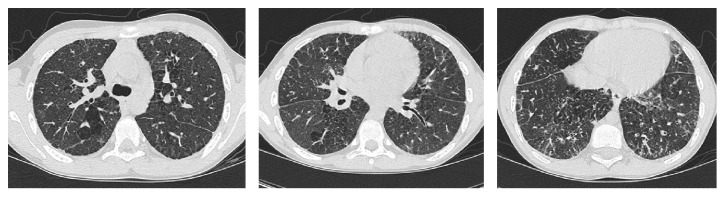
CT of chest showed area of emphysematous changes, several small lung cysts, patchy ground-glass opacity, interlobular septal thickening, and bronchiectasis.
